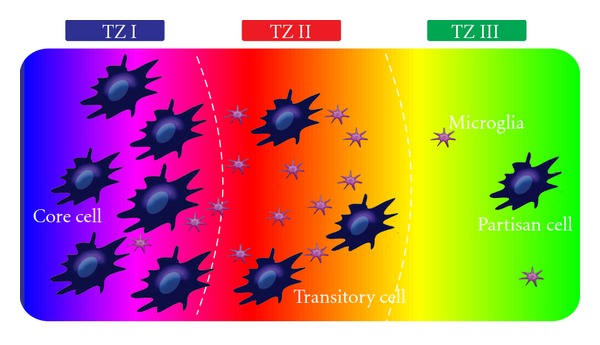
The brain tumor microenvironment, heterogeneous tumor zones and MIF actions. Conceptual framework depicting the metabolic and immune cell complexity of malignant brain tumors, (glioblastomas, GBM) is given as a simplified model classifying the tumor into three distinct tumor zones (TZ1–TZ3). Tumor Zone 1 (TZ1) consists of the main tumor—bulk and core glioma cells, corresponding to contrast enhancing regions in MRI images. MIF is mainly produced in TZ1 and secreted into the extracellular space. TZ2 represents the area of perifocal edema, which is characterized by its specific proangiogenic microenvironment and presence of transitory glioma cells. In addition, this tumor zone shows pronounced accumulation of microglial cells, which also infiltrate TZ1. TZ3 is the most awkward zone for therapeutic intervention, since this tumor zone consists mainly of healthy brain parenchyma. However, isolated glioma-initiating cells termed partisan cells colonize TZ3 and are most probably responsible for tumor recurrence following surgery. TZ2 is probably biologically most active, influencing TZ1 and TZ3 by tumor-derived metabolites impacting the immune system, angiogenesis, and cell fate.
